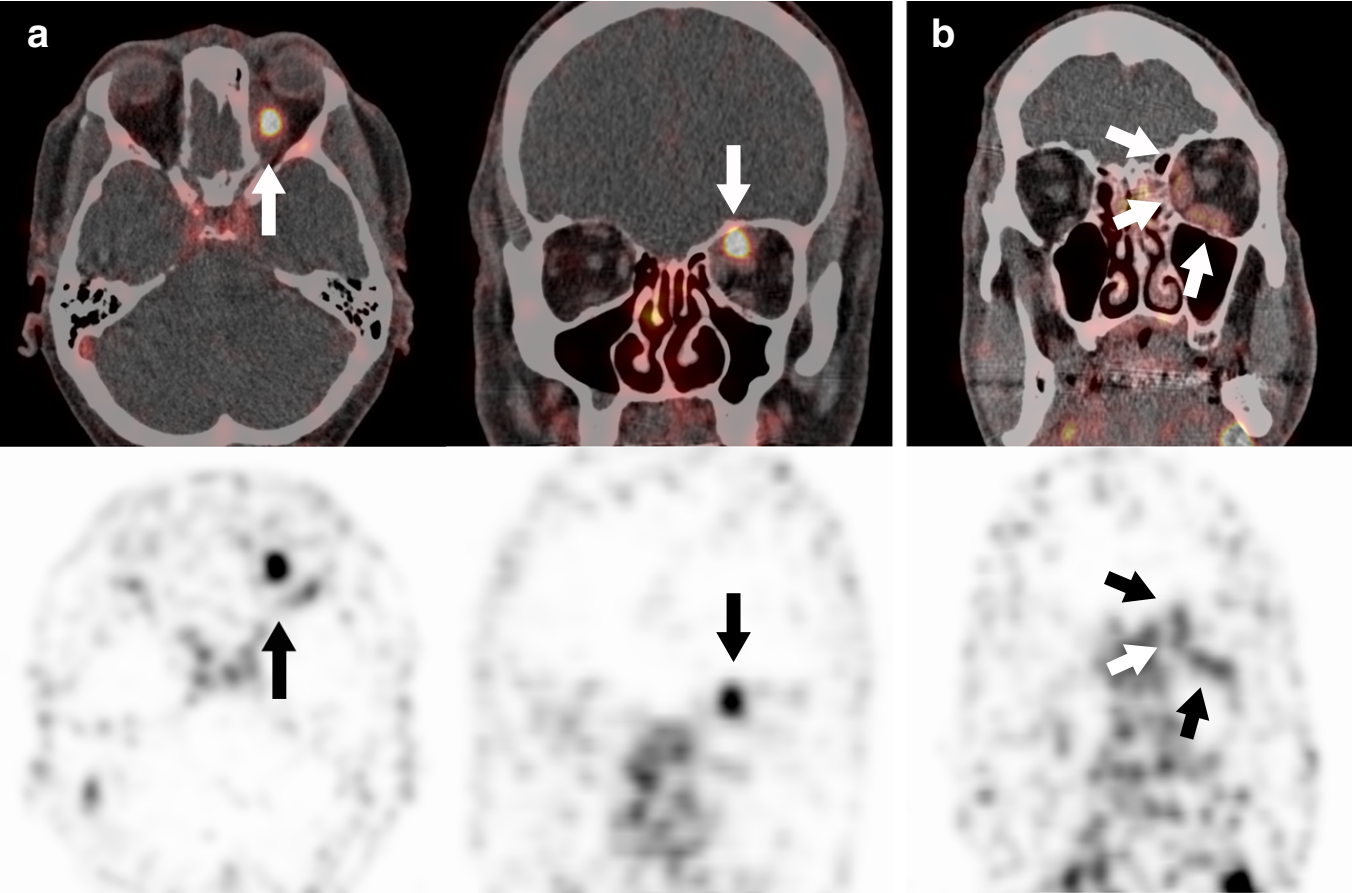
Examples of 89Zr-rituximab PET/CT uptake. a Strong uptake in fusion image and PET-only image for the axial and coronal planes (arrows point at the lesion, SUVmax> 2.0; case 6). b Moderate uptake in fusion image and PET-only image (arrows point at affected muscles, SUVmax 1.0–2.0, case 5)
